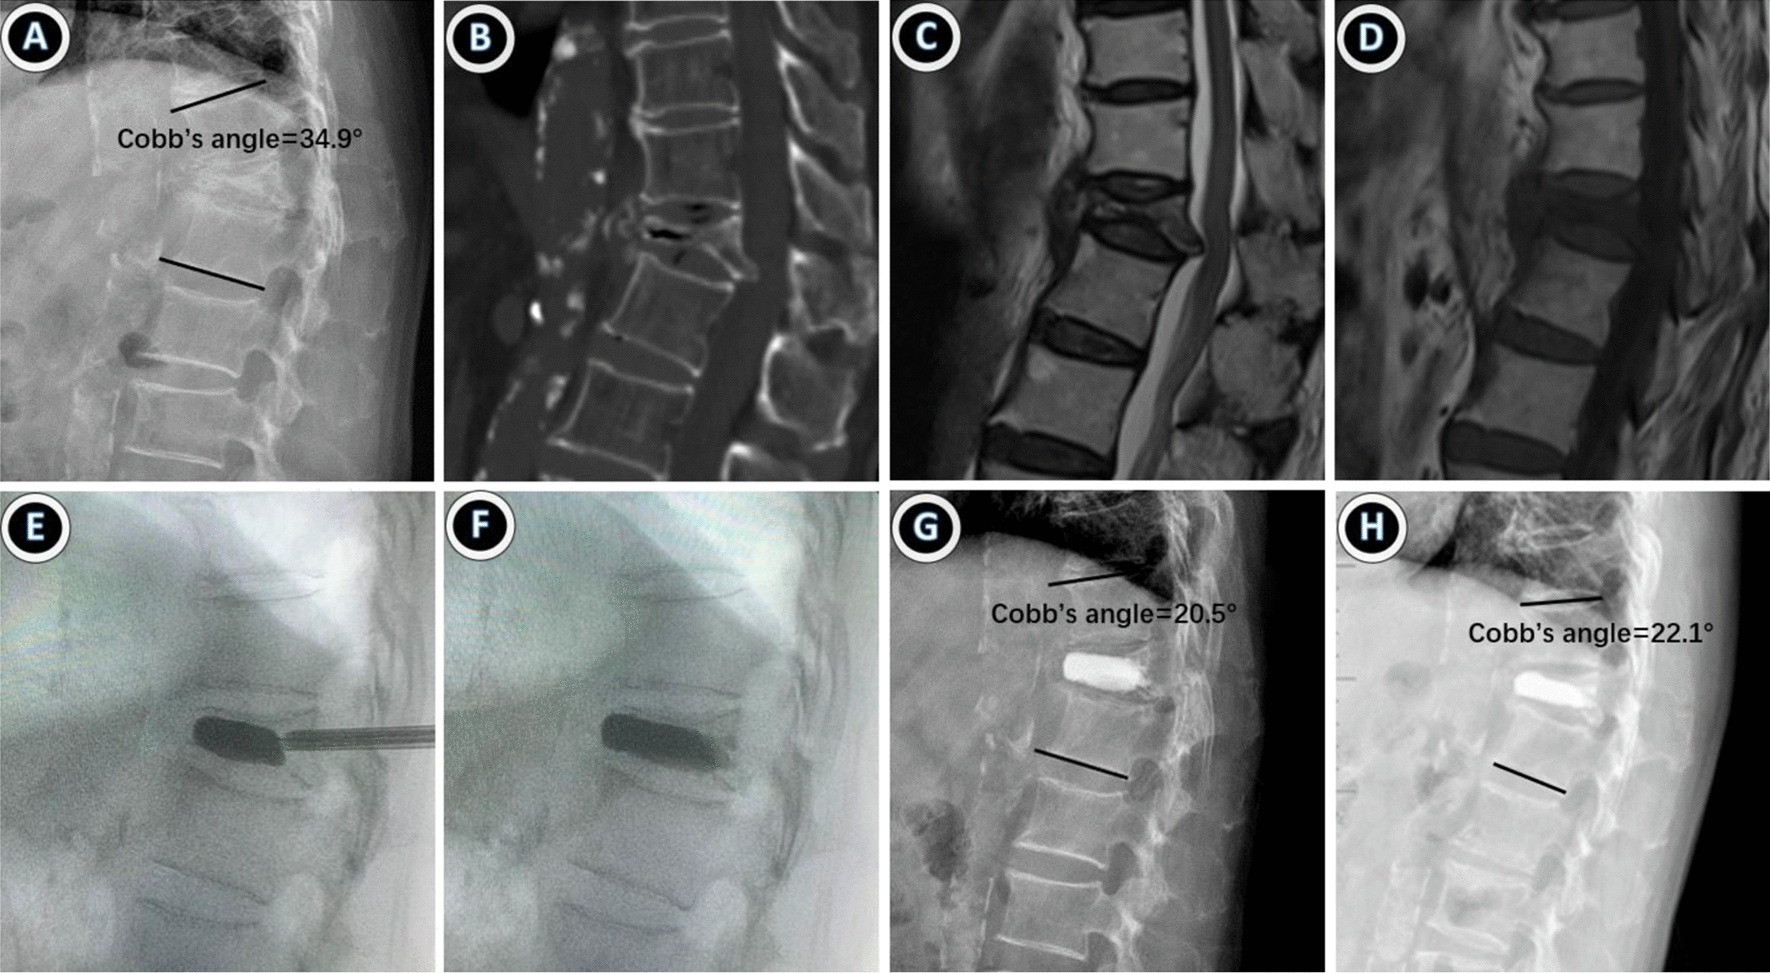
An 84-year-old woman who had T12 sOVCF with nonunion was treated with PKP. A–D The preoperative X-ray, CT and MRI showed a severe osteoporotic vertebral compression fracture with nonunion. E, F The compressed vertebral body was completely dilated during surgery using balloons and then bone cement was injected. G, H The postoperative and final follow-up X-ray displayed the vertebral height and the Cobb’s angle was well recovered
